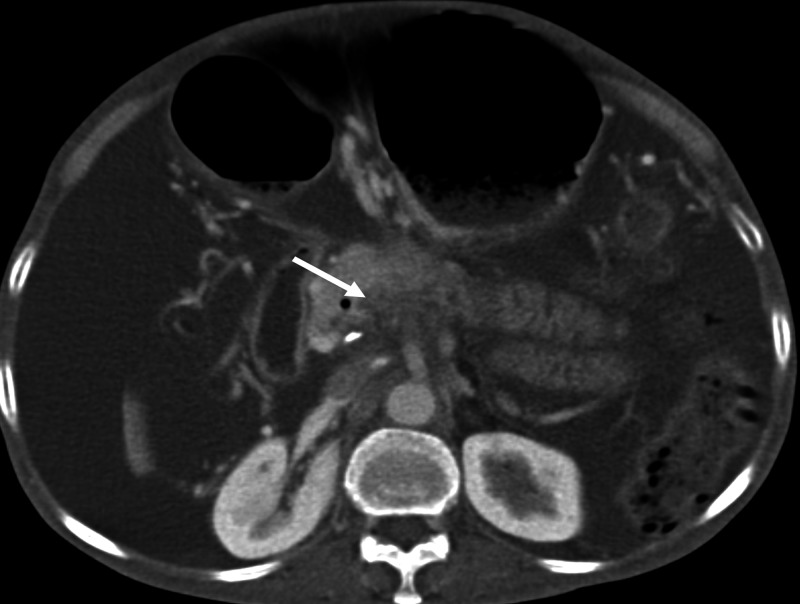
A computed tomography scan of the abdomen demonstrating thrombosed splenic and superior mesenteric veins with collaterals around.
